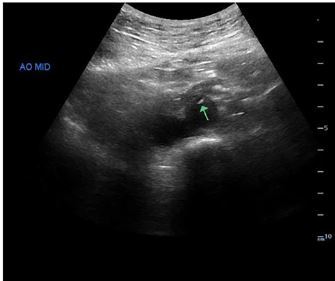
Grayscale ultrasound demonstrating intimal flap (arrow) in mid-aorta.
